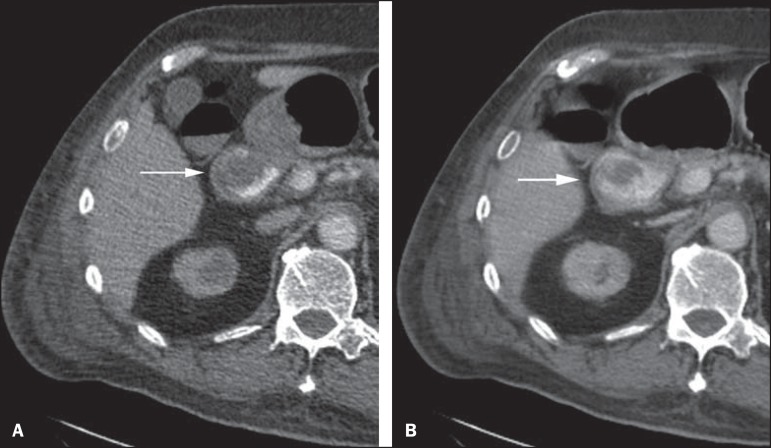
Male, 65-year-old patient presenting with hematemesis. CT angiography
